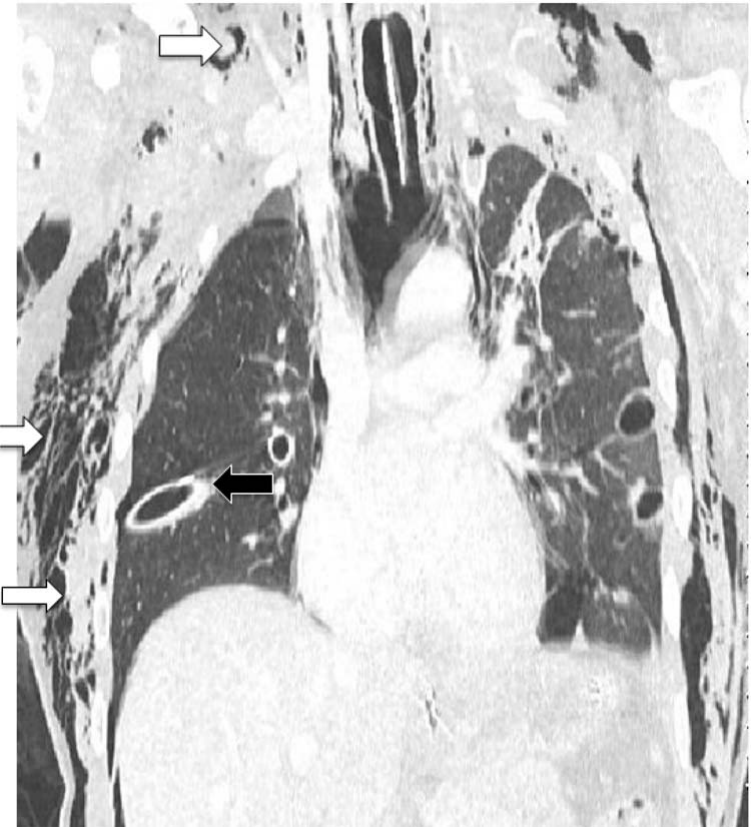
CT chest showing chest tubes in situ (black arrow) and soft tissue emphysema (white arrows).
